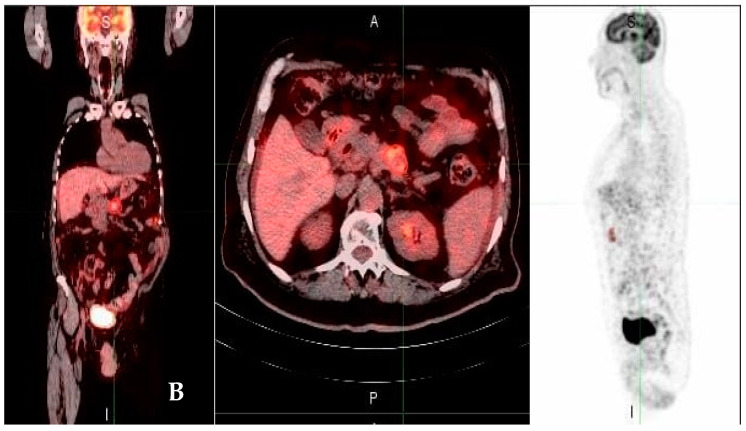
A 66-year-old man was diagnosed with stage II pancreatic adenocarcinoma (T3N0M0). PET/CT scans performed at baseline reveal minor metabolic activity (yellow regions, (A,B); maximum standardized uptake value, 3.7) consistent with primary pancreatic mass; projection of maximal intensity PET imaging demonstrates 33 mL of tumor glycolytic activity without signs of metastatic involvement (pancreatic body mass).
